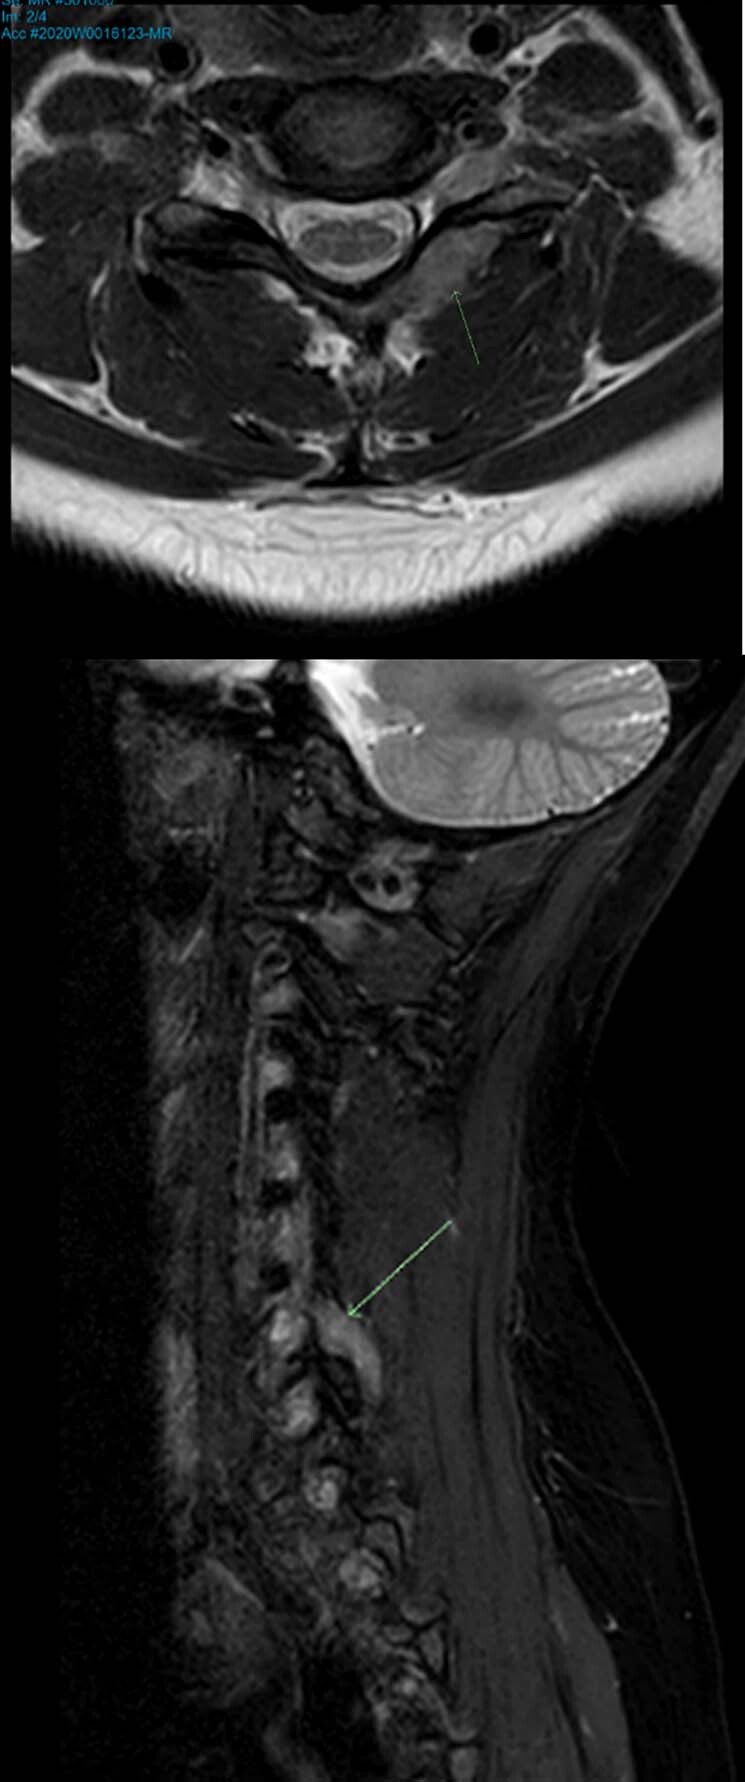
Case 2 MRI scan. There was a 19 mm intermediate T1/T2 signal area in the left posterior perivertebral tissues between the C6 and C7 lamina. This extended to the margin of the left facet joint.
